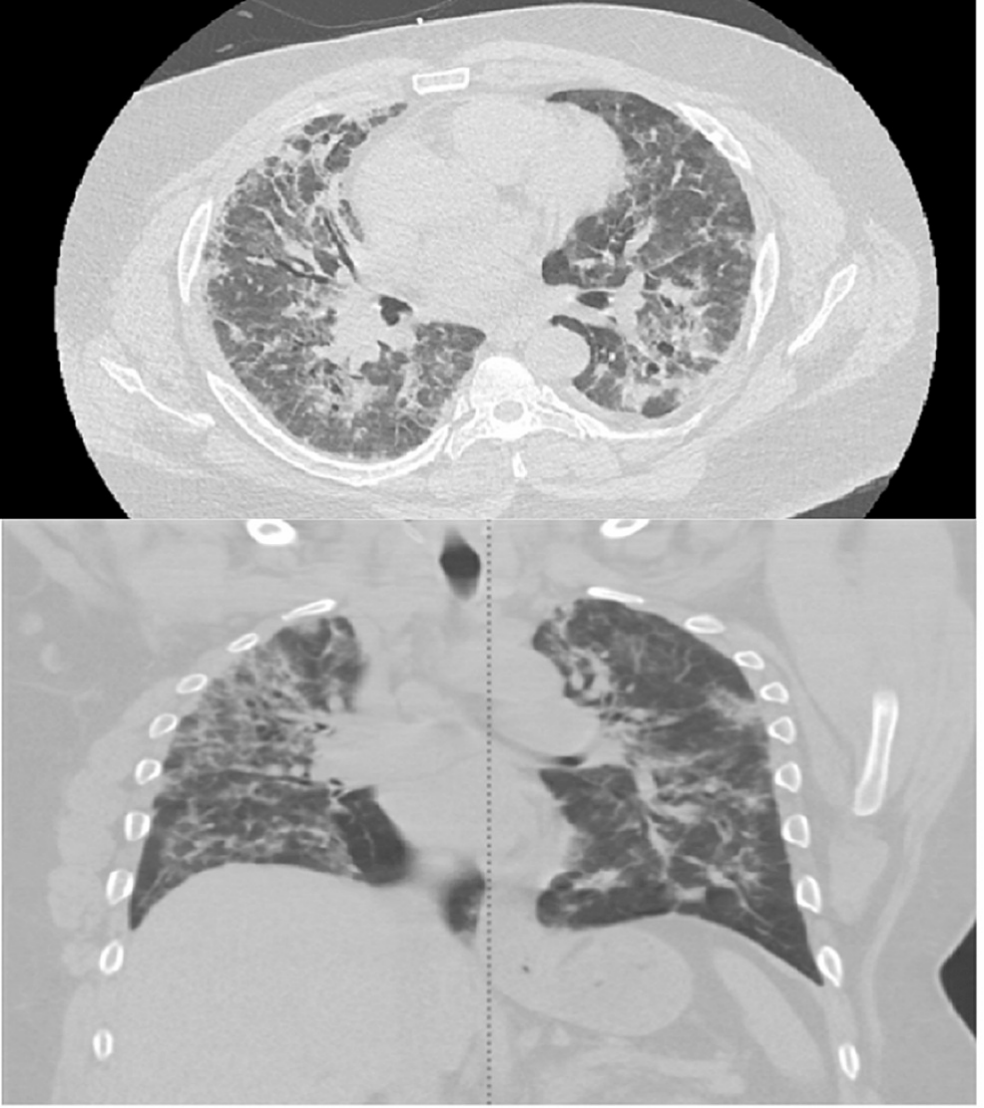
Axial lung window CT image and coronal CT lung window image demonstrate peripheral areas of consolidation, with faint adjacent areas of ground glass opacification The findings are nonspecific and cryptogenic organizing pneumonia and idiopathic eosinophilic pneumonia remain in the differential radiologically.
